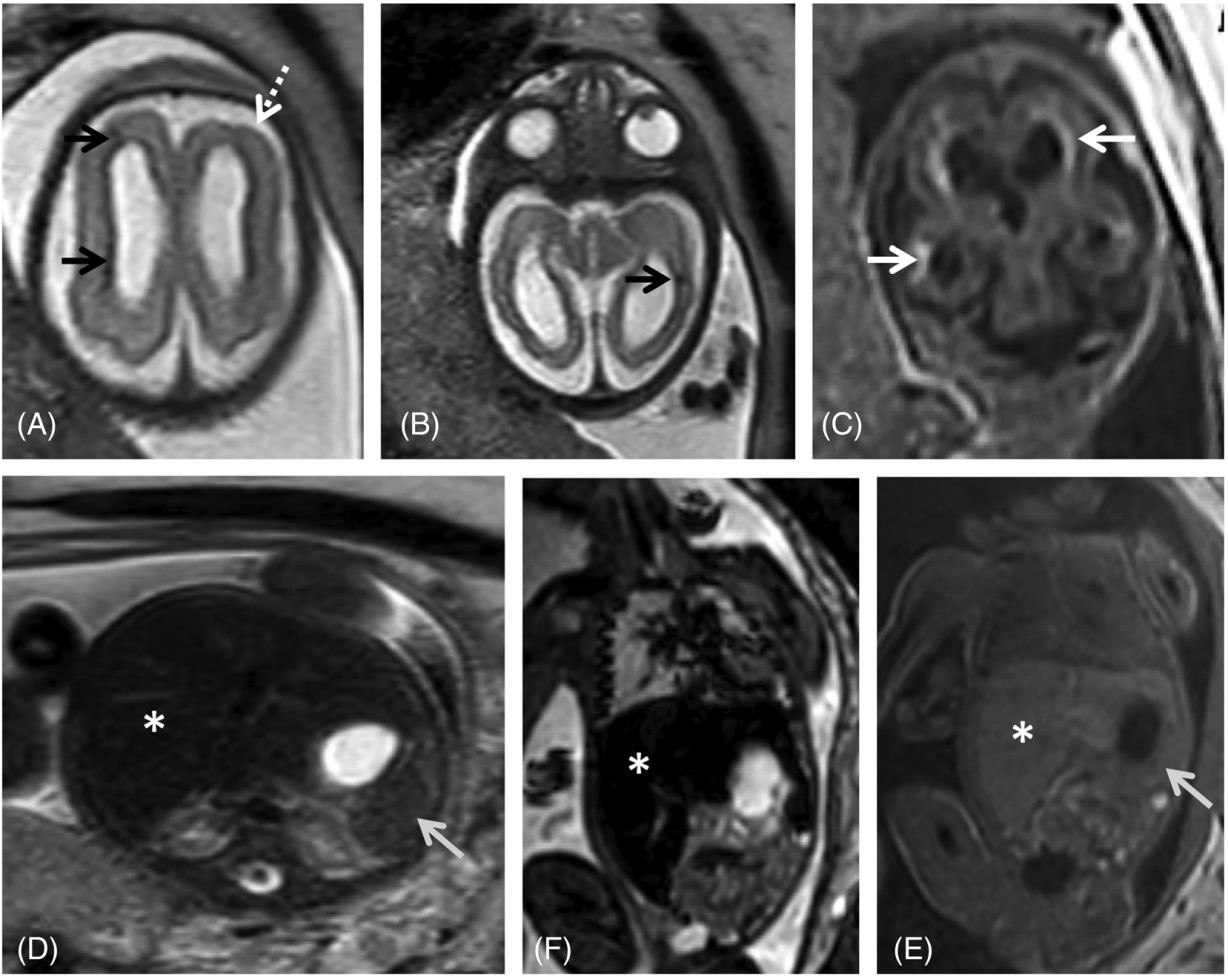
Fetal magnetic resonance imaging (MRI) at 29 gestational weeks referred for microcephaly and splenomegaly. There is parenchymal loss, with global thinning of the cerebral mantle and consequent bilateral ventriculomegaly. Small areas of focal signal anomaly can be detected on T2WI (A, B, black arrows), as well as frontal polymicrogyria (A, white dashed arrow). Calcifications can be identified on T1WI (c, white arrows). Enlarged spleen (gray arrow) and liver (asterisk) can be identified on T2W steady state free precession (D), echo planar imaging (E) and T1W images (F), with slight signal intensity anomaly on the latter
